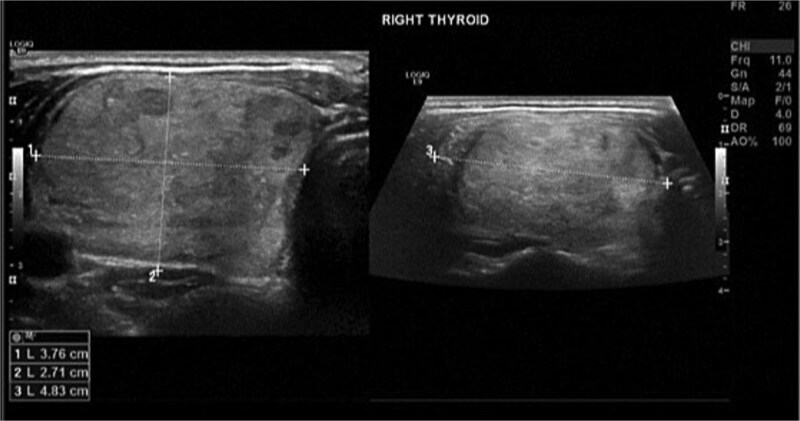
Ultrasound shows the dimensions of the right thyroid lobe measured at 38 × 27×48 mm.
